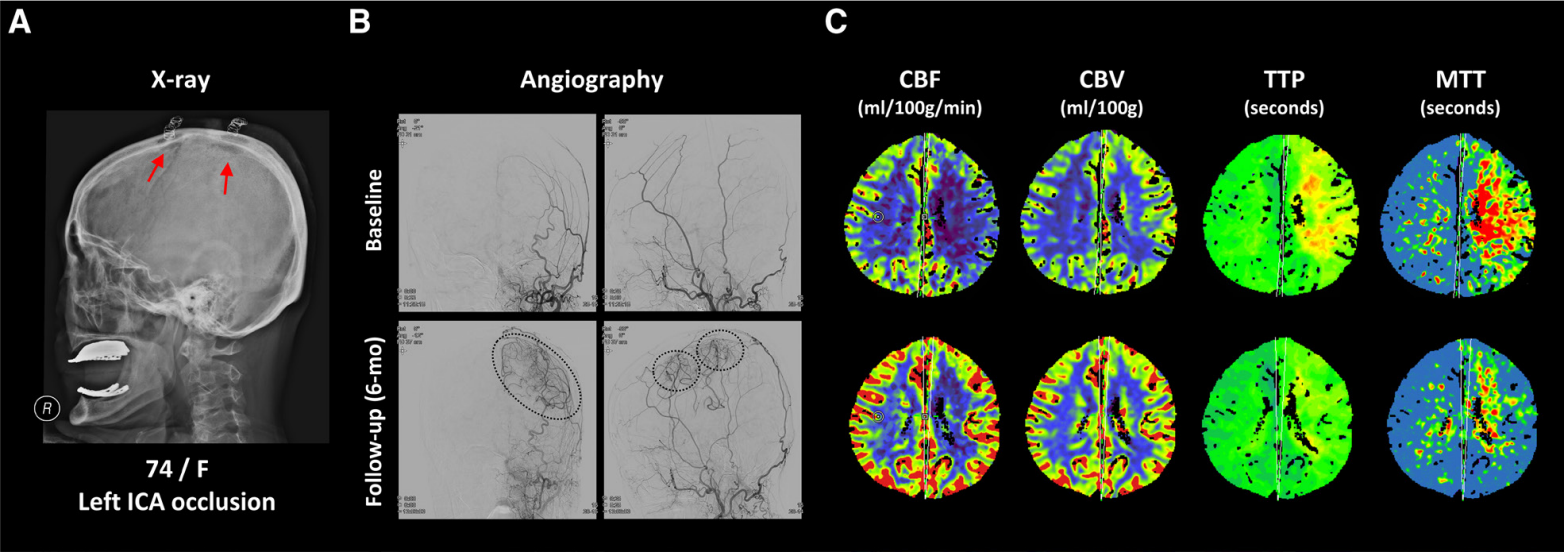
Representative case showing good transdural collaterals after combination therapy. A, Skull radiography after combination therapy in a 74-year-old woman with left internal carotid artery occlusion. Red arrows indicate the multiple burr hole (MBH) region. B, Angiography and (C) perfusion CT (cerebral blood flow [CBF], cerebral blood volume [CBV], time-to-peak [TTP], and mean transit time [MTT]) at baseline and 6 mo after combination therapy. The dotted ellipses indicate the transdurally developed arteriogenesis in the MBH regions.
